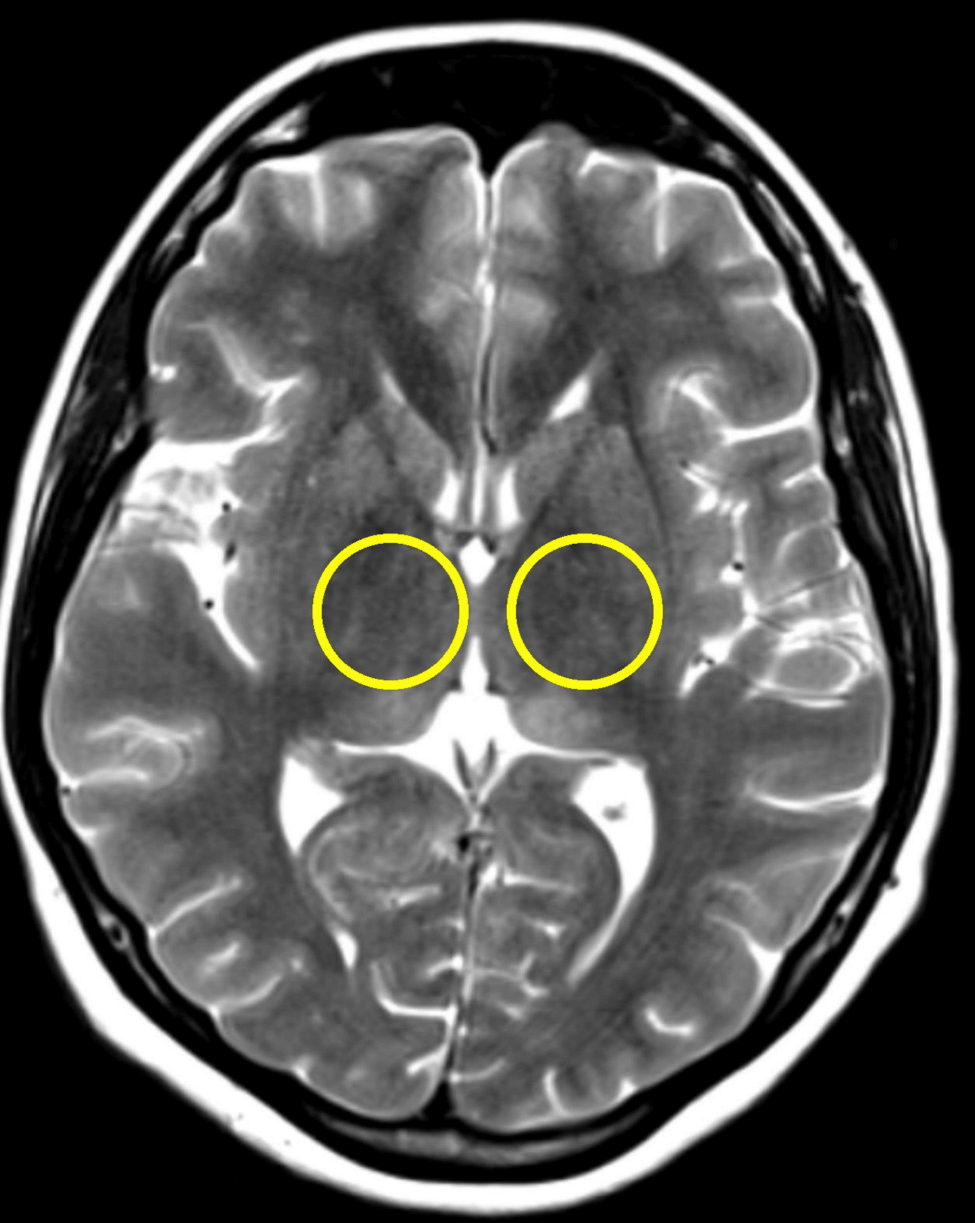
Axial T2-Weighted MRI Brain Image Axial T2-weighted MRI demonstrating bilateral thalamic hyperintensity, more prominent on T2 sequences. These findings are consistent with inflammatory or autoimmune encephalitis and align with reported imaging patterns in immune checkpoint inhibitor–related neurotoxicity.
